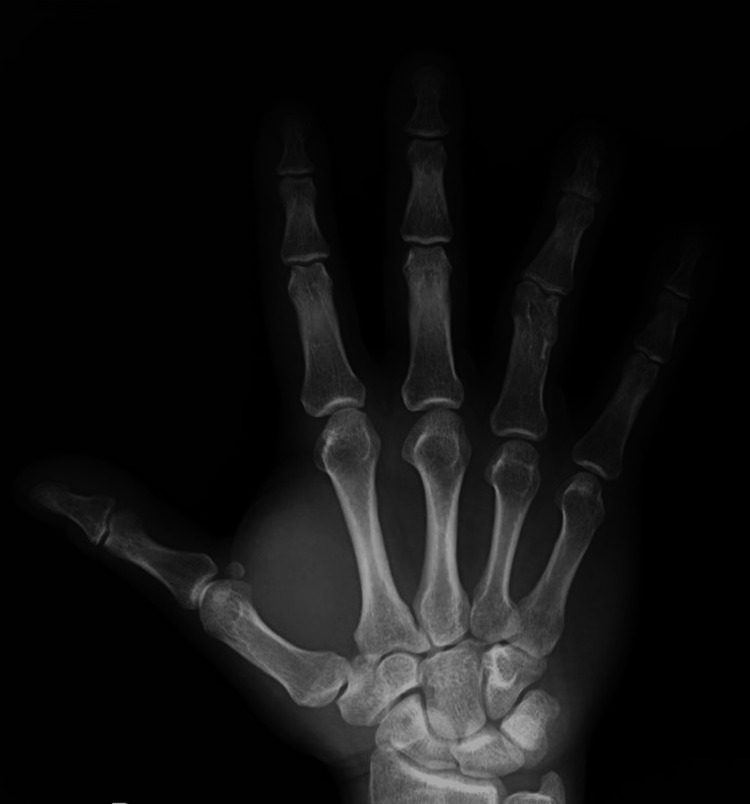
X-ray of the right hand in AP projection, three years after the initial treatment with Kirschner wires AP: anteroposterior
